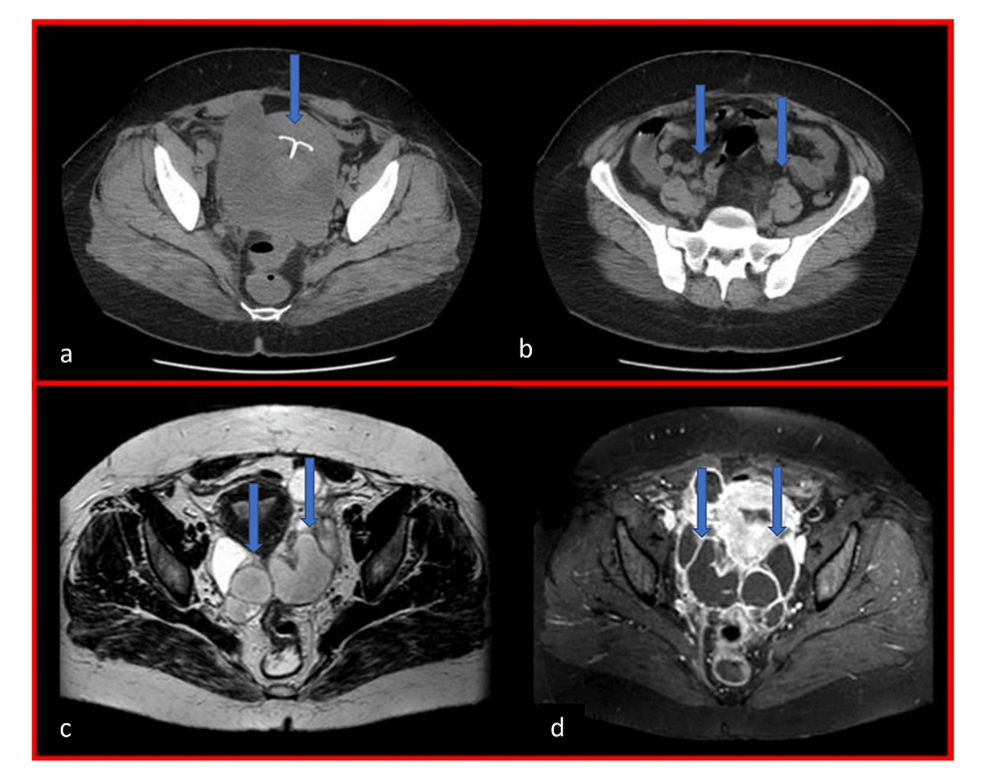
CT scan transverse sections showing IUCD, uterus, and overdistended tubes (a) Arrow showing intrauterine contraceptive device (IUCD) inside the uterus. (b-d) Arrows pointing to overdistended tubes on both sides.
